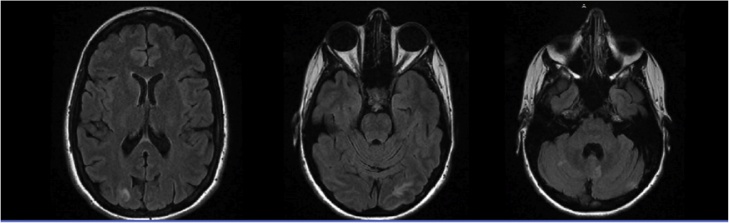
MRI imaging showing the patchy T2 and diffusion hyperintensity in the occipital loves and adjacent cerebellar hemisphere.
